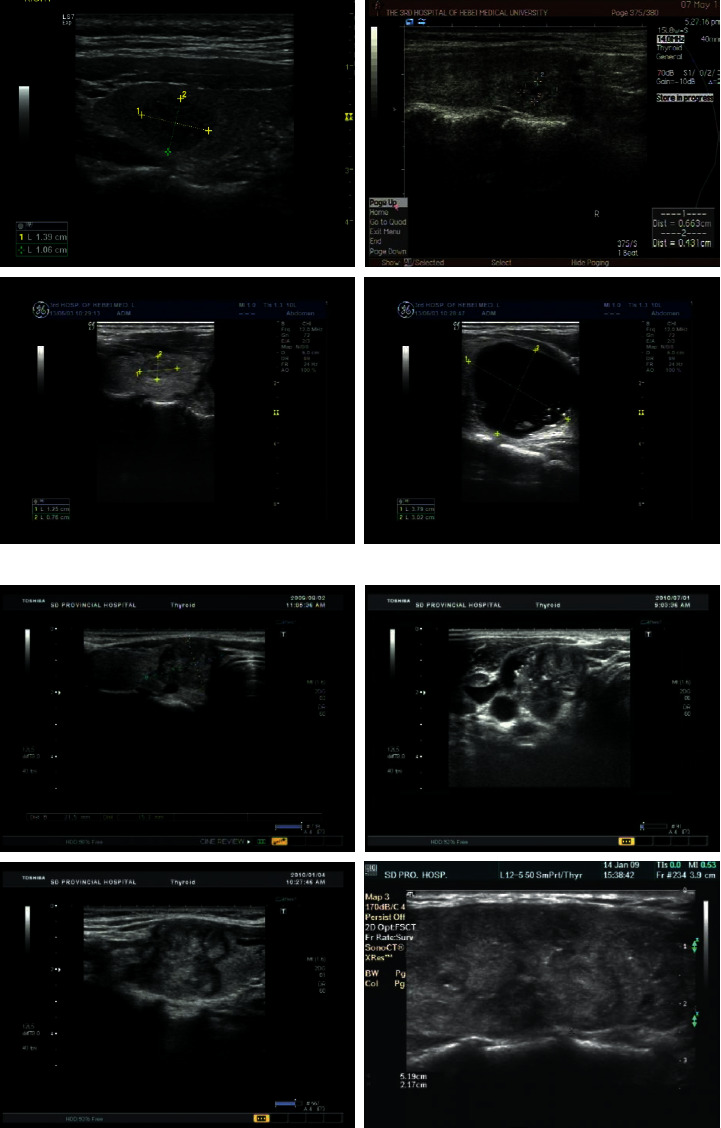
Examples of the original ultrasound image with thyroid nodules. (a) The thyroid nodules of the upper four images are benign. (b) The thyroid nodules of the lower four images are malignant.
